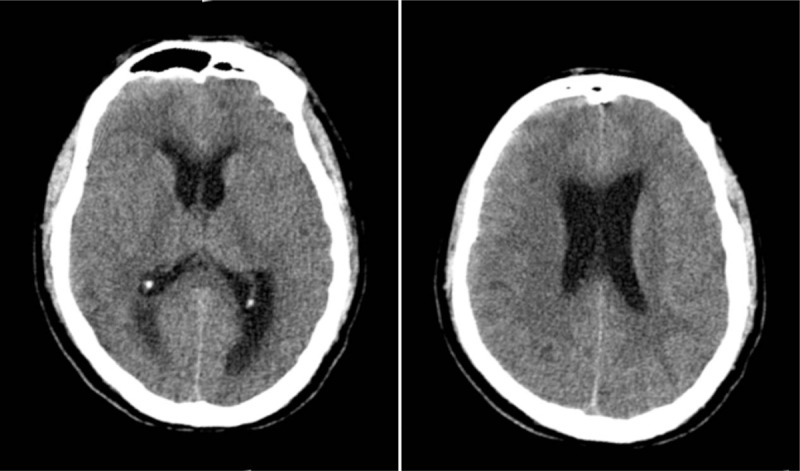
Head computed tomography showing cerebral edema and hydrocephalus.
